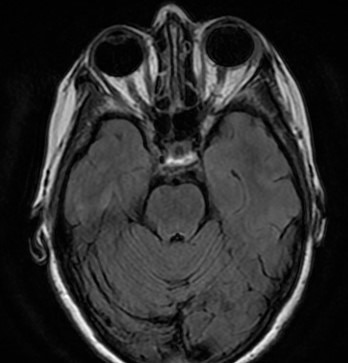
Orbital magnetic resonance image. Posterior scleral thickening and proptosis of the LE
